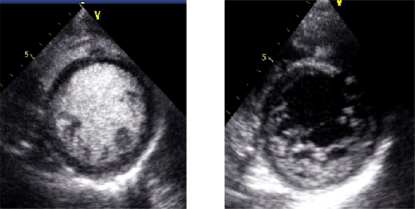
Parasternal short axis view with and without contrast in a patient presenting with acute heart failure. The contrast injection allows a better separation of the compacted (yellow dots) and non-compacted layer and to apply the criteria of diagnosis in isolated non-compaction of the left ventricle.
